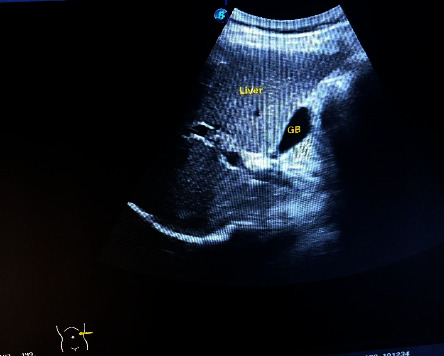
An abdominal ultrasound of the left upper quadrant reveals the gallbladder (GB) and liver on the left side, as opposed to their typical anatomical location.
